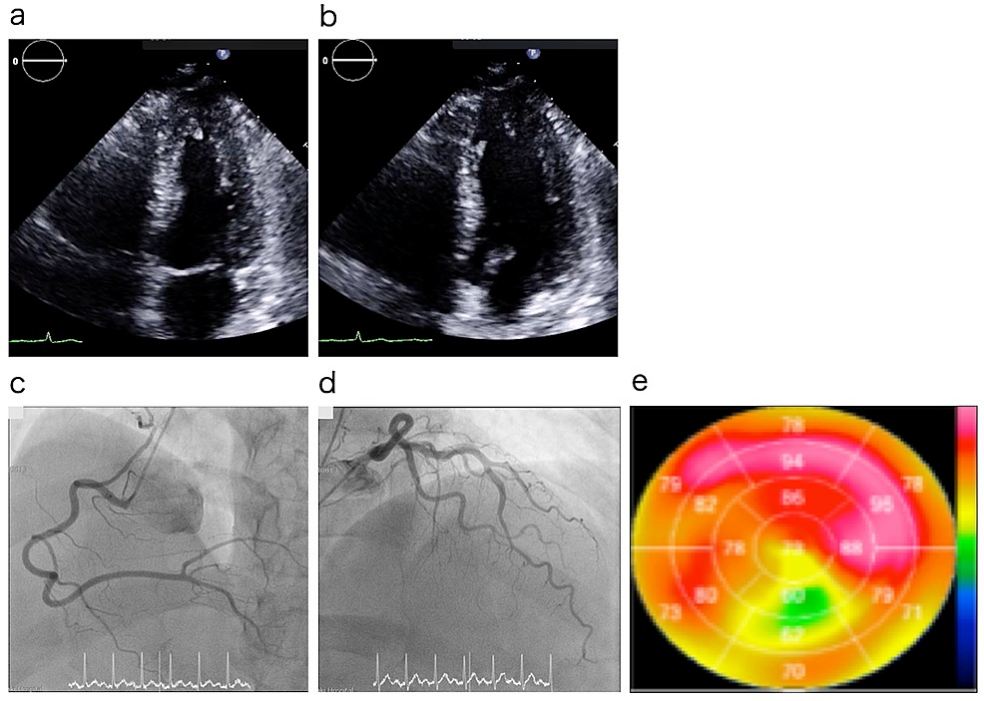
Transthoracic echocardiogram, coronary angiography and 123I- metaiodobenzylguanidine (MIBG) myocardial scintigraphy. Transthoracic echocardiogram performed immediately after the recovery from ventricular tachycardia showing hypokinesia of the left ventricular apex. Apical approach, four-chamber view: (a) systolic phase; (b) diastolic phase. Coronary angiography (CAG) performed three days after ventricular tachycardia and 123I-metaiodobenzylguanidine (MIBG) myocardial scintigraphy performed 10 days after discharge. CAG revealed no cardiac contractility and no coronary arterial stenosis: (c) right coronary artery; (d) left coronary artery. (e) Bull’s eye image. MIBG accumulation decreased mainly in the apex.
